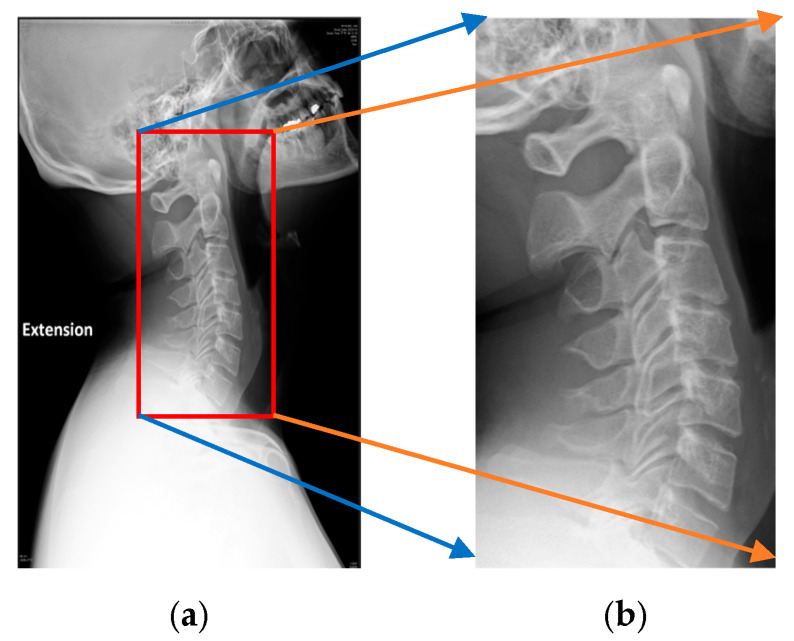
The result of the X-ray cervical spine localization. (a) Original cervical spine radiography. (b) The image after algorithm processing.
